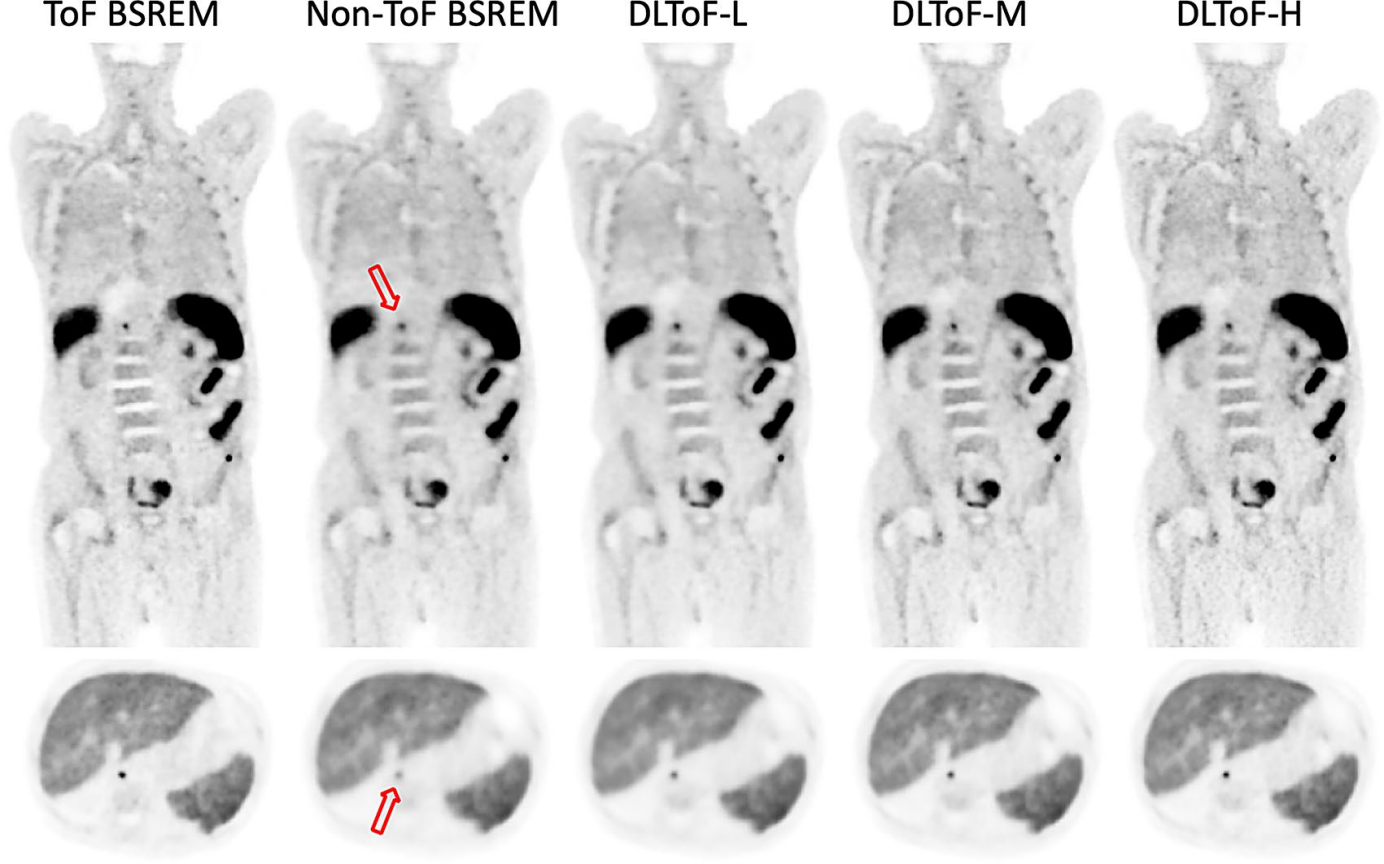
DL-ToF enhancement of a ^18^F-PSMA test subject with a BMI of 23.5 kg/m2 with an injected activity of 346.7 MBq of scanned on DMI PET/CT scanner. Arrows point to lesions with lower conspicuity in non-ToF BSREM. Display window: 0–5 (top) and 0–15 (bottom) SUV
